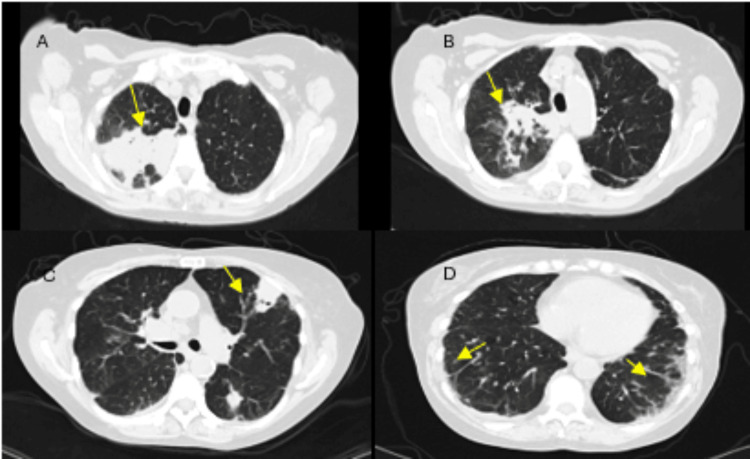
CT of the chest without contrast showing multifocal pulmonary opacities (arrows) in the right upper lobe (A, B), left upper lobe (C), and fibrotic changes at the lung bases (D). The right and left upper lobe opacities contain several bubbles of air, which may indicate a cavitary process.
